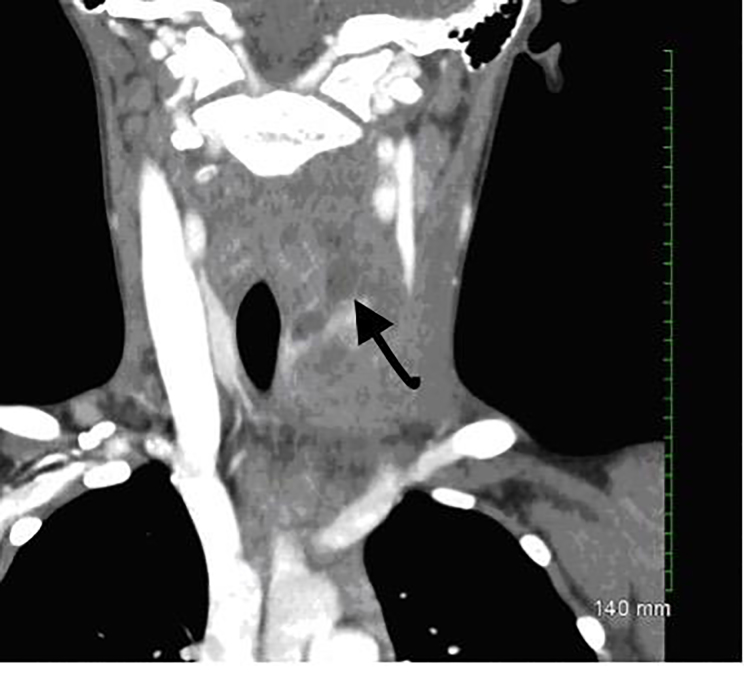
Coronal computed tomography of the neck demonstrating multiloculated heterogeneous fluid (arrow) in the left thyroid lobe
